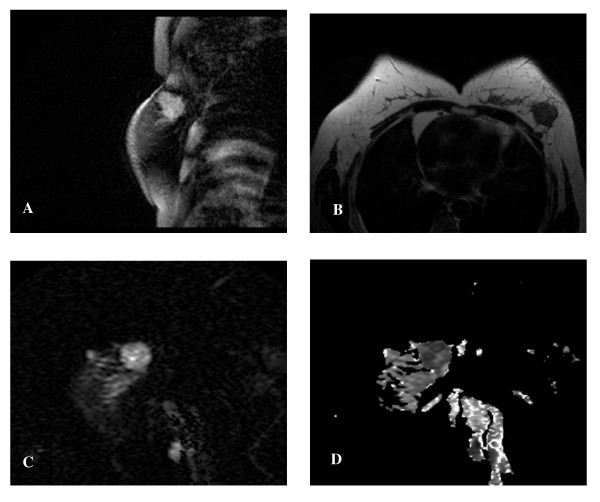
45-year-old woman with IDC in the left breast. A: WATS T1-weighted sagittal scan showed irregularly mass in the left breast with hyperintense signal. B: T2-weighted transverse scan showed the lesion was hypointense with spicule sign. C: EPI-DWI sagittal scan showed lesion with slightly inhomogeneity hyperintense in DWI. D: Inhomogeneity hypointense was found in ADC map (b = 500) and the ADC was 0.94 ± 0.19 ± 10^-3^mm^2^/s.
